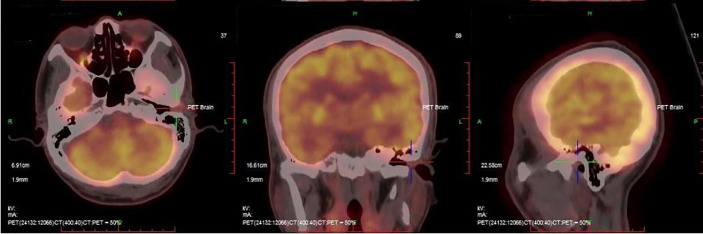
PET-CT scan of the whole body demonstrated the ﬂuorodeoxyglucose (FDG)-avid mass (maximum standard uptake volume (SUVmax 2.6).
